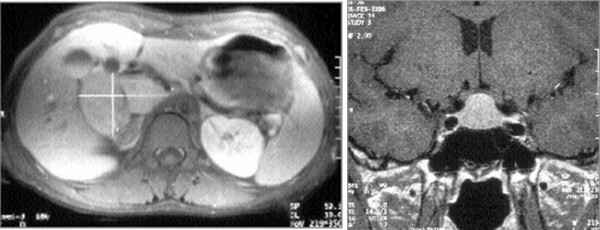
Acromegaly due to ectopic secretion of GHRH by a pancreatic tumor, metastasized to the liver (left) responsible for a pituitary hyperplasia, visible on pituitary MRI (right) and stimulation of normal somatotroph cells, leading to GH hypersecretion and acromegaly.
